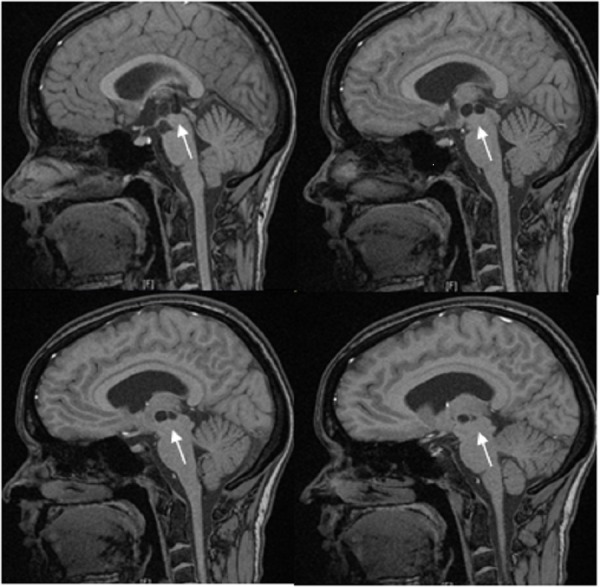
Patient imaging five years prior to initial presentation T1 weighted, non-contrast, sagittal brain MRI demonstrating a multi-loculated third ventricular cystic structure (arrows).
